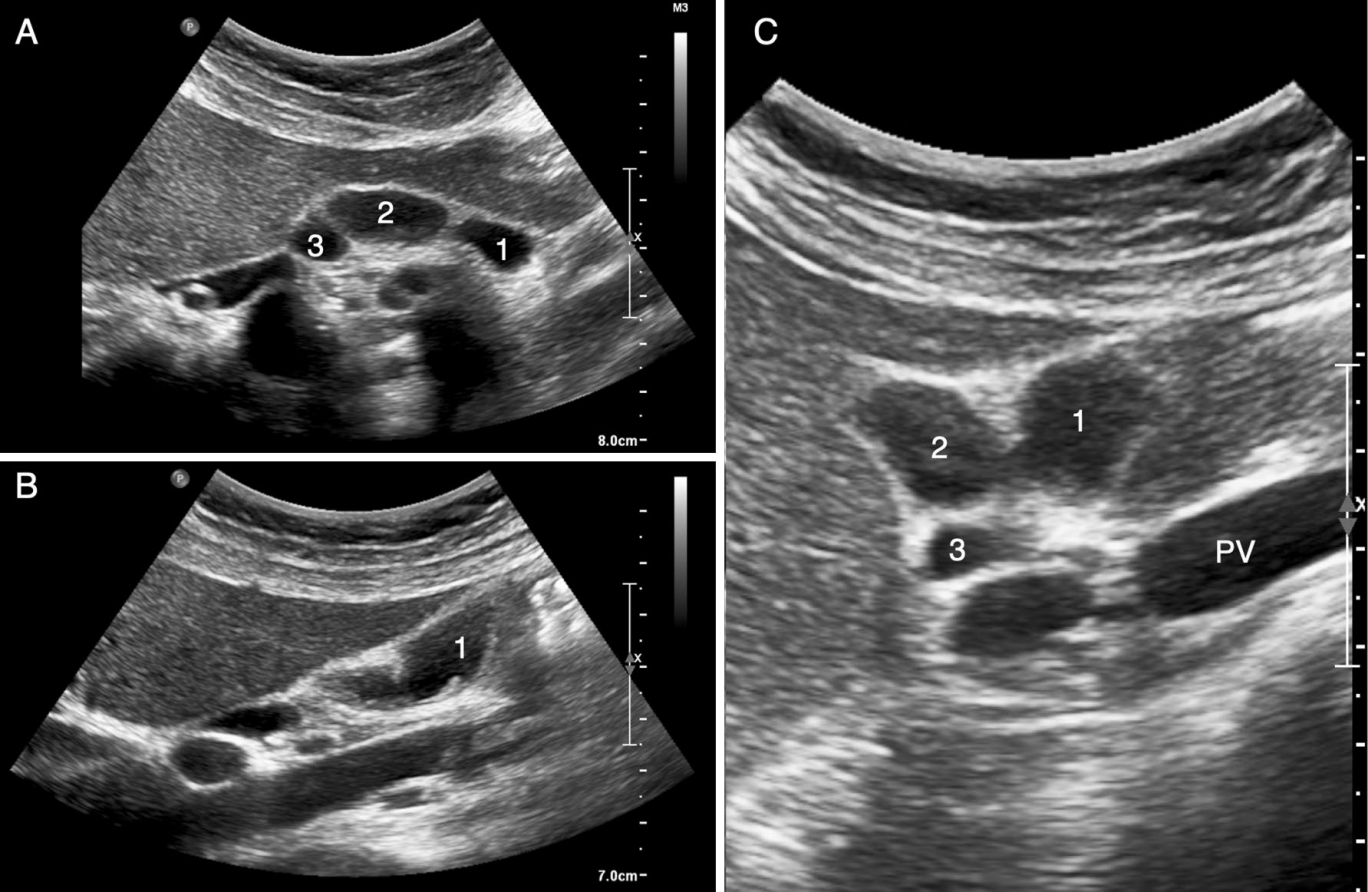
Ultrasound examination showed three different gallbladders (numbers 1, 2, 3 in panels A and C) in the cholecystic
